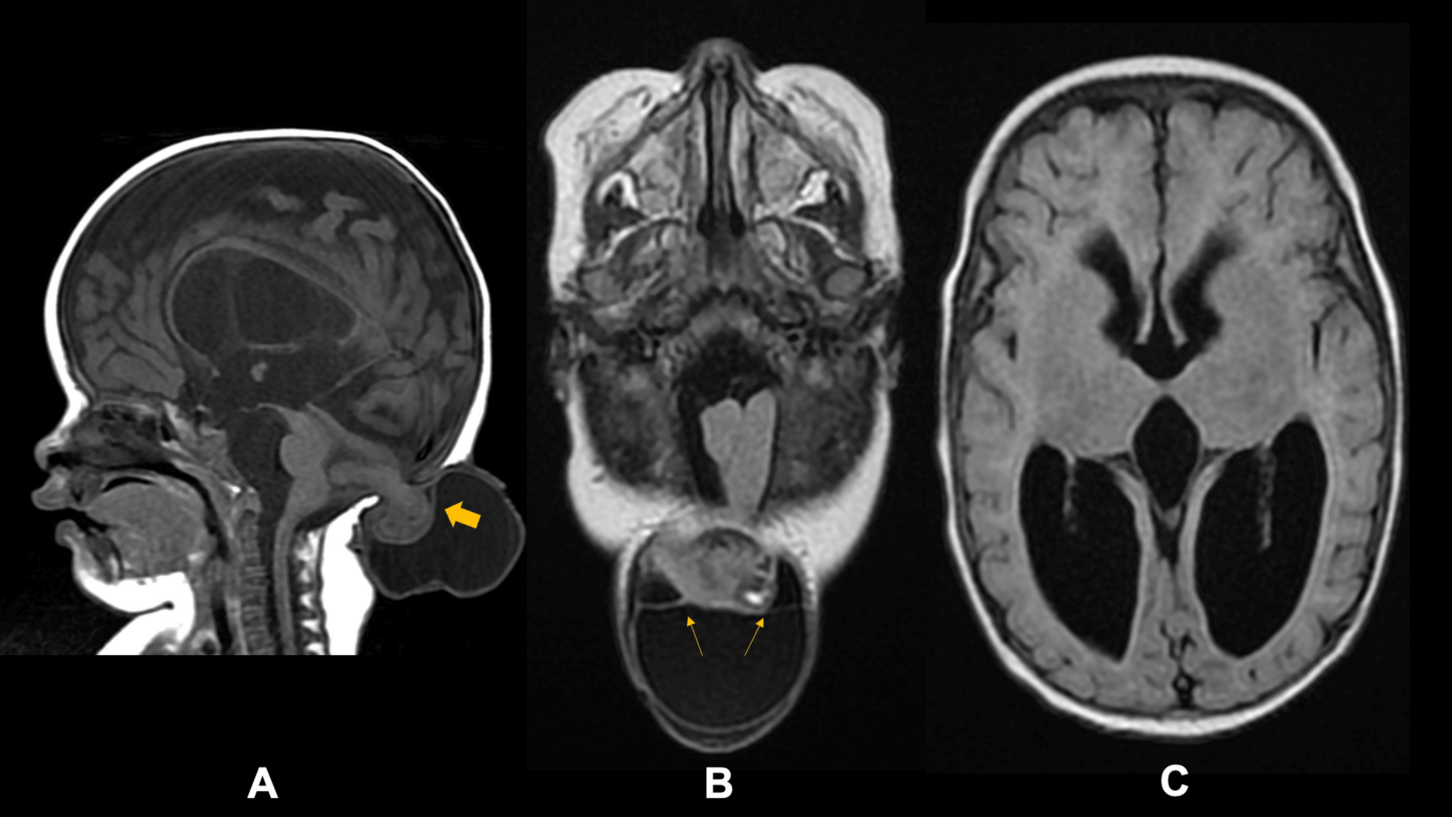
Multiplanar and multisequential MR scans of the brain were obtained using the 1.5 Tesla GE Signa Voyager Scanner (GE Healthcare, Chicago, Illinois, US) Cranial MR imaging showed extracranial herniation of SF and some of the posterior fossa structures composed of the right cerebellar hemisphere (dark arrow) and portion of the vermis (A) measuring approximately 2.5 x 2.5 x 2.1 cm (CCWAP; 4.4 x 4.5 x 5.2 cm including the SF component), through a midline low occipital bone defect (diameter of 1.1 cm). There is associated herniation of the meninges with some septations (thin arrow) and folding of the dura (B). There is a subsequent posterior traction effect on the cerebellar vermis and brainstem, with elongation of the fourth ventricle and aqueduct of Sylvius. There is consequent dilatation of the third and lateral ventricles (C). There is thinning of the corpus callosum and compression of the cerebral hemispheres. *Abbreviations: *MRI: Magnetic resonance imaging; SF: spinal fluid; SWI: susceptibility-weighted imaging; GRE: gradient-recall echo; FLAIR: fluid-attenuated inversion recovery *Legend: *A: T1 sagittal; B and C: T2 FLAIR axial
